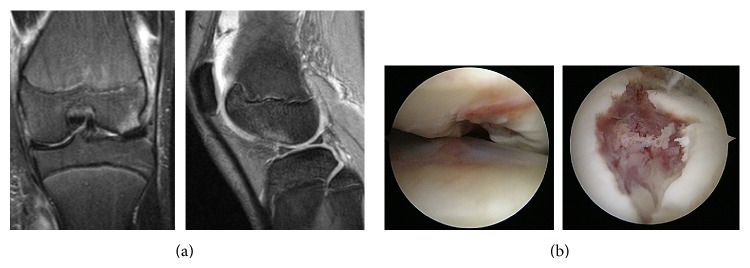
Additional findings in Patient 2. (a) T2-weighted MRI, showing a bone bruise in the lateral femoral condyle, as well as a cartilage defect of the lateral femoral condyle. (b) During extension of the knee joint, an osteochondral defect was observed in the joint surface of the weight-bearing portion of the lateral femoral condyle (left). At 90° flexion of the knee joint, a 10 × 15-mm osteochondral defect was clearly observed (right).
